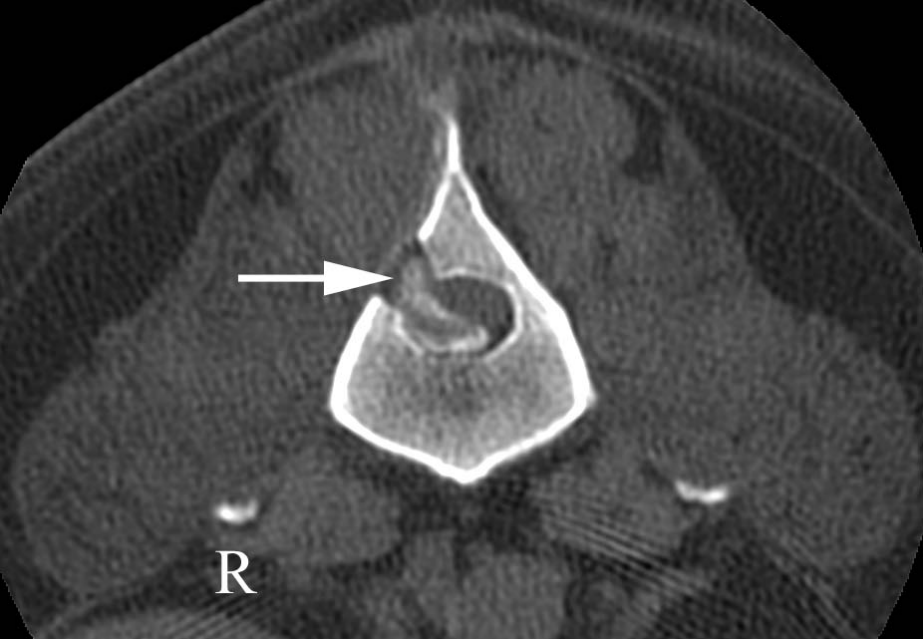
CT scan in bone window through the center of L2 eleven days after right sided hemilaminectomy. Calcified material (arrow) in the spinal canal is compressing the spinal cord dorsally and laterally towards the left. These findings suggest a recurrent disc extrusion at L1-2 that was confirmed at surgery.
